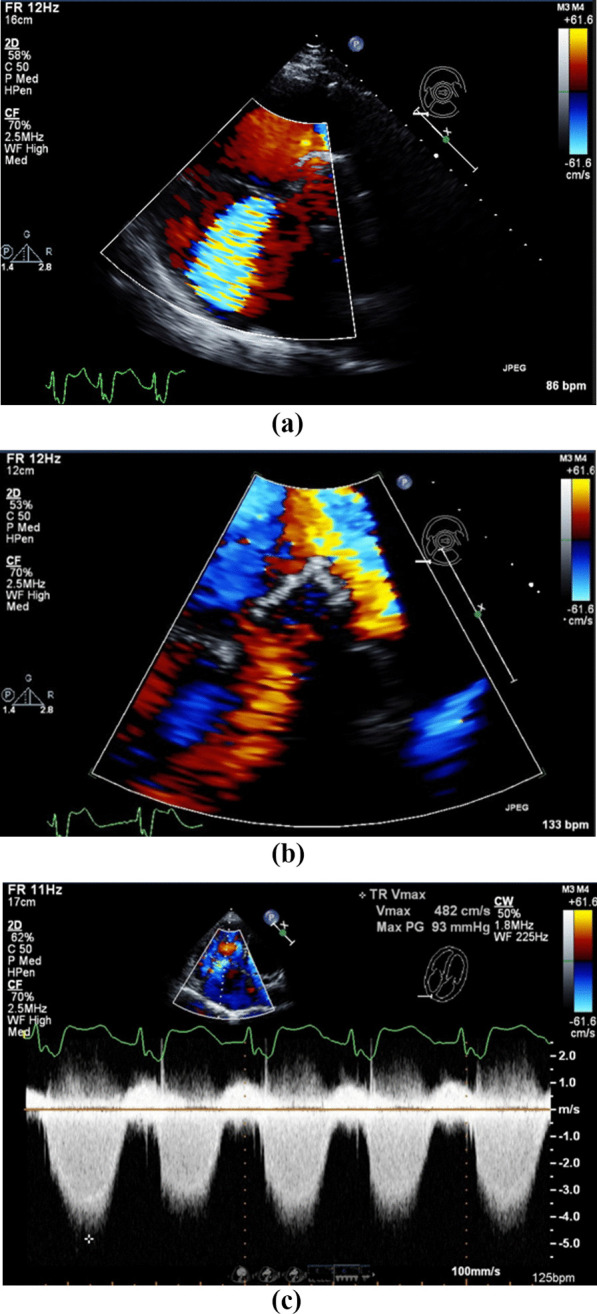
Transthoracic echocardiography revealing severe pulmonic regurgitation. a Parasternal short axis of the tricuspid valve inflow tract showing severe tricuspid regurgitation by color flow doppler. b Parasternal short-axis view with broad color flow jet covering the total diameter of the right ventricular outflow tract and showing severe pulmonic regurgitation. c Continuous-wave doppler through the tricuspid valve showing a peak gradient of 93 mmHg suggesting severe pulmonary hypertension in the apical view
